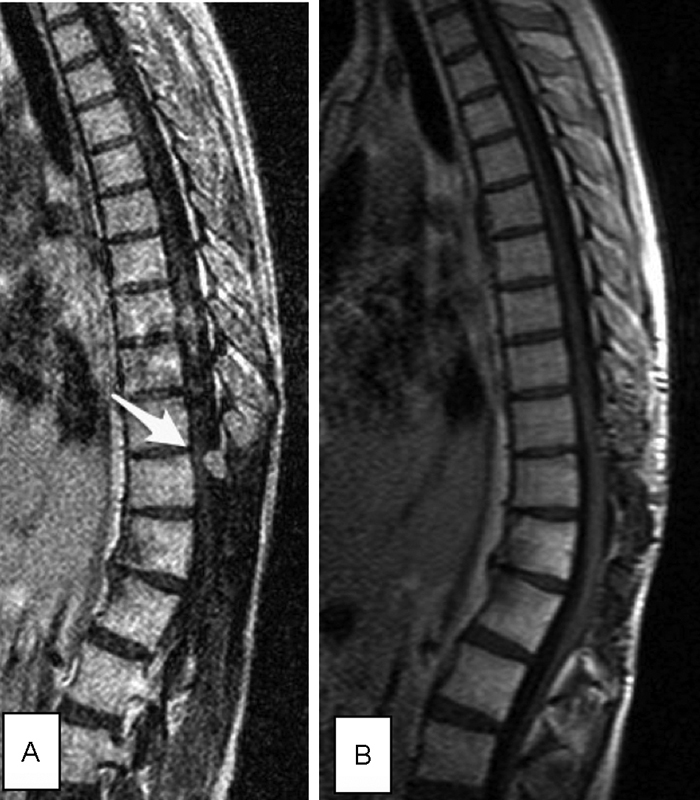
Second recurrence of the tumor in 2008 in MR imaging. (
